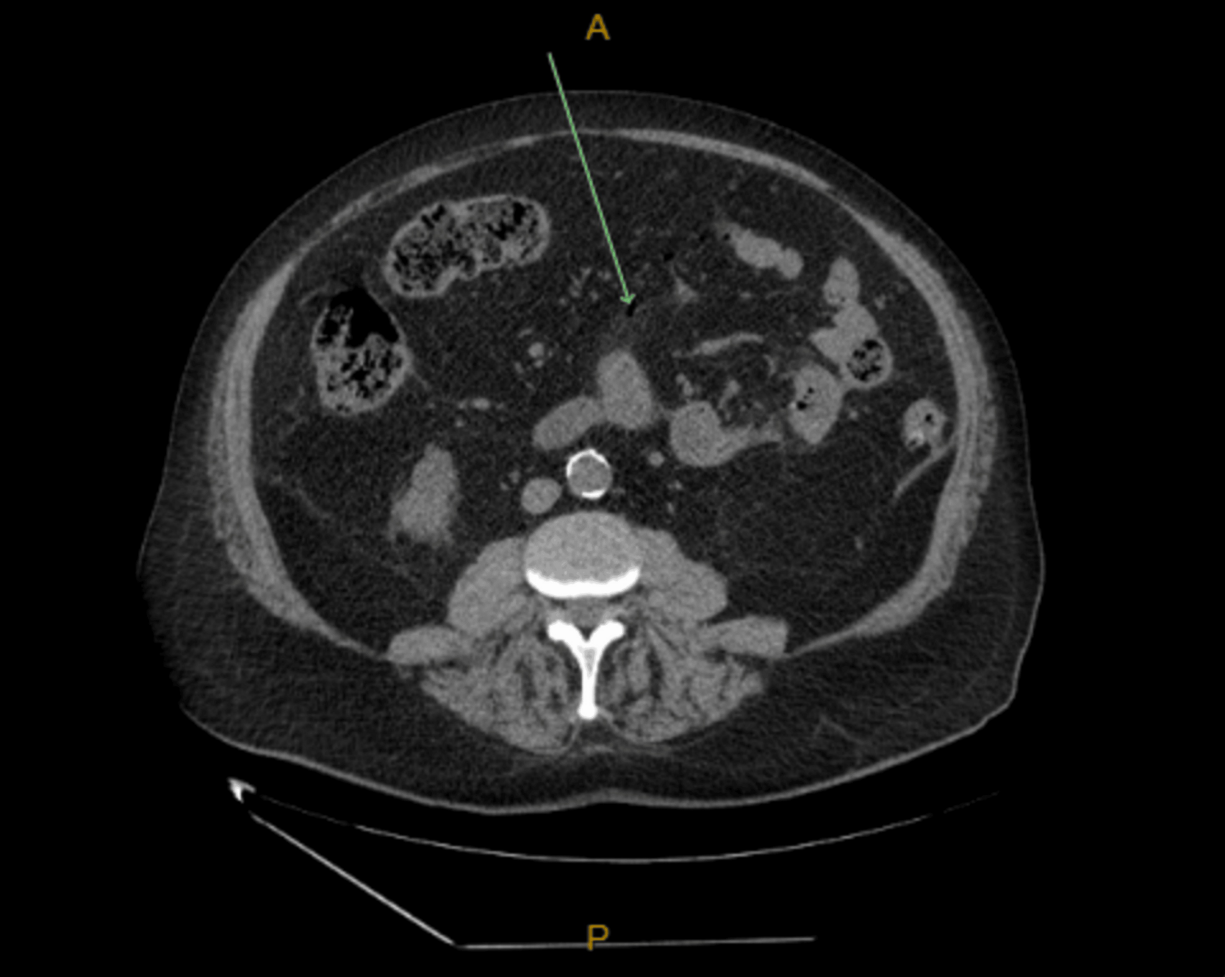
Axial computed tomography (CT) image of the abdomen with oral and intravenous contrast showing sigmoid diverticulosis
